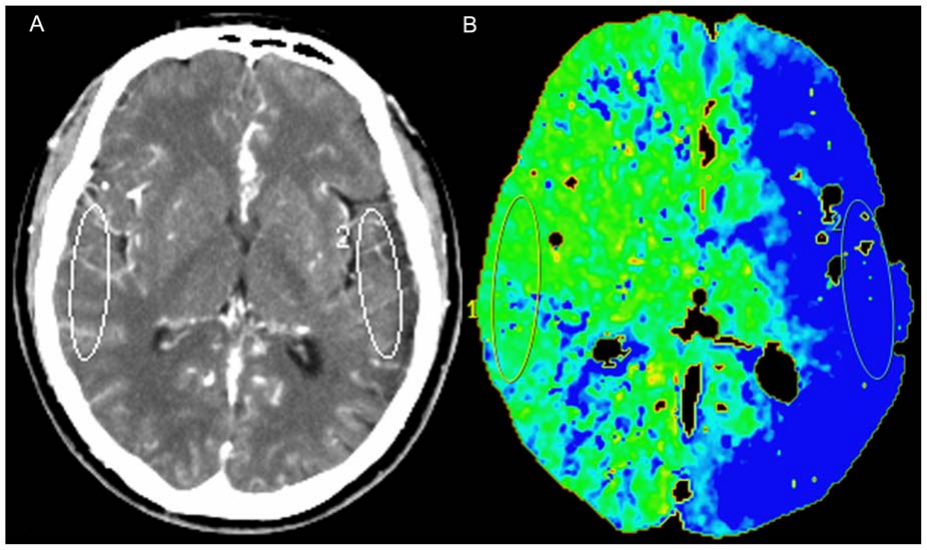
Regions of interest (ROIs) were placed on cortical regions in the middle cerebral artery territory. ROIs were drew in (A) the reference CT image, and (B) vessel-removed mean transit time (MTT) map.
